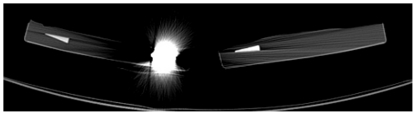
Streak artifact produced by Amalcap in CT.
